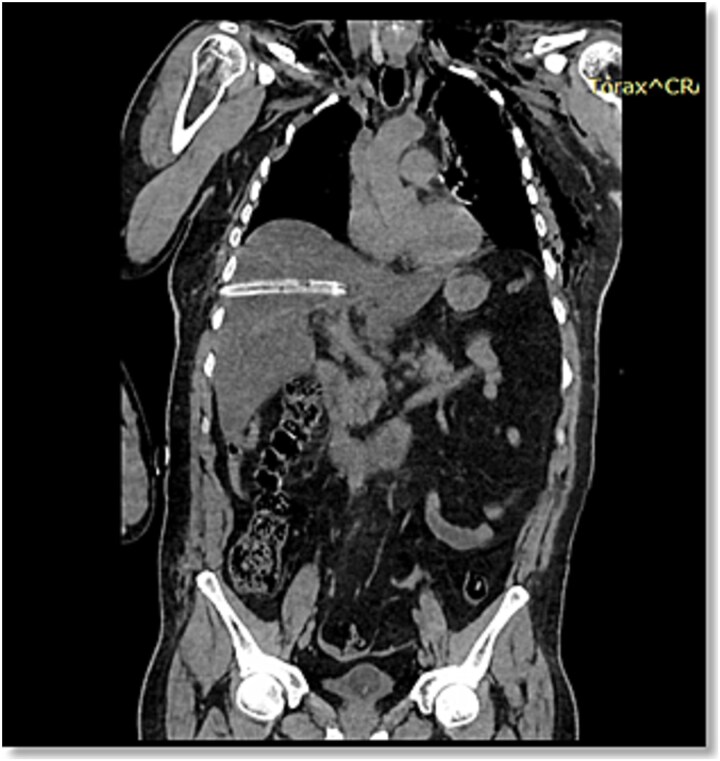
Non-contrast CT of the chest, abdomen, and pelvis, coronal view, demonstrating a chest tube inserted through the seventh intercostal space penetrating hepatic segments VII, VIII, and I. No free intraperitoneal fluid is identified.
